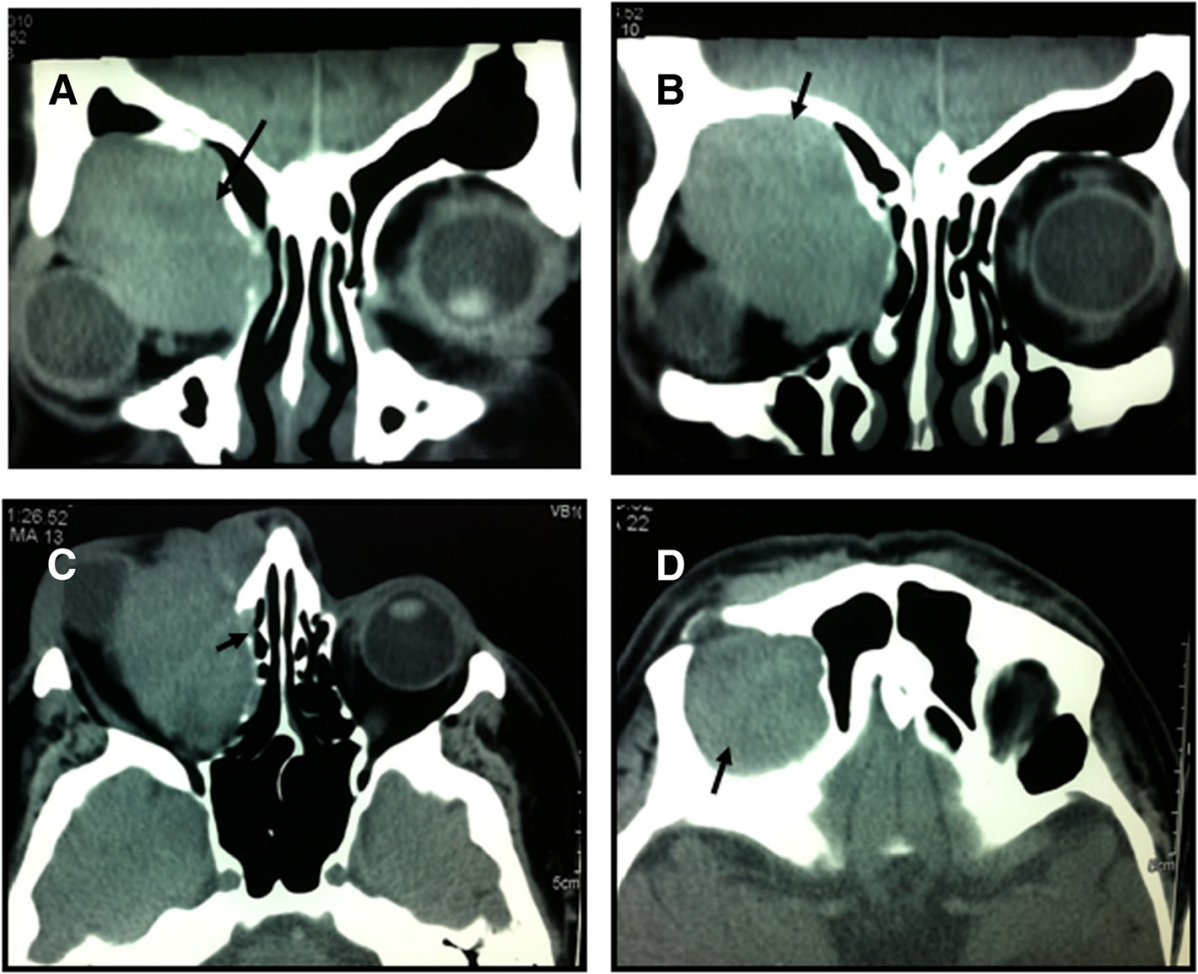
Orbital computed tomographic scans revealing dermatofibrosarcoma protuberans. (A) and (B) Coronal images. (C) and (D) Axial images. These computed tomographic scans reveal a well-defined soft tissue mass along the superomedial aspect of the right orbit (arrows in A and B). The mass is causing pressure remodeling of the right lamina papyracea and ensuing fractional annihilation of the right ethmoidal sinus (arrow in C). The mass is also causing pressure remodeling of the right orbital roof, extending cranially (arrow in D).
